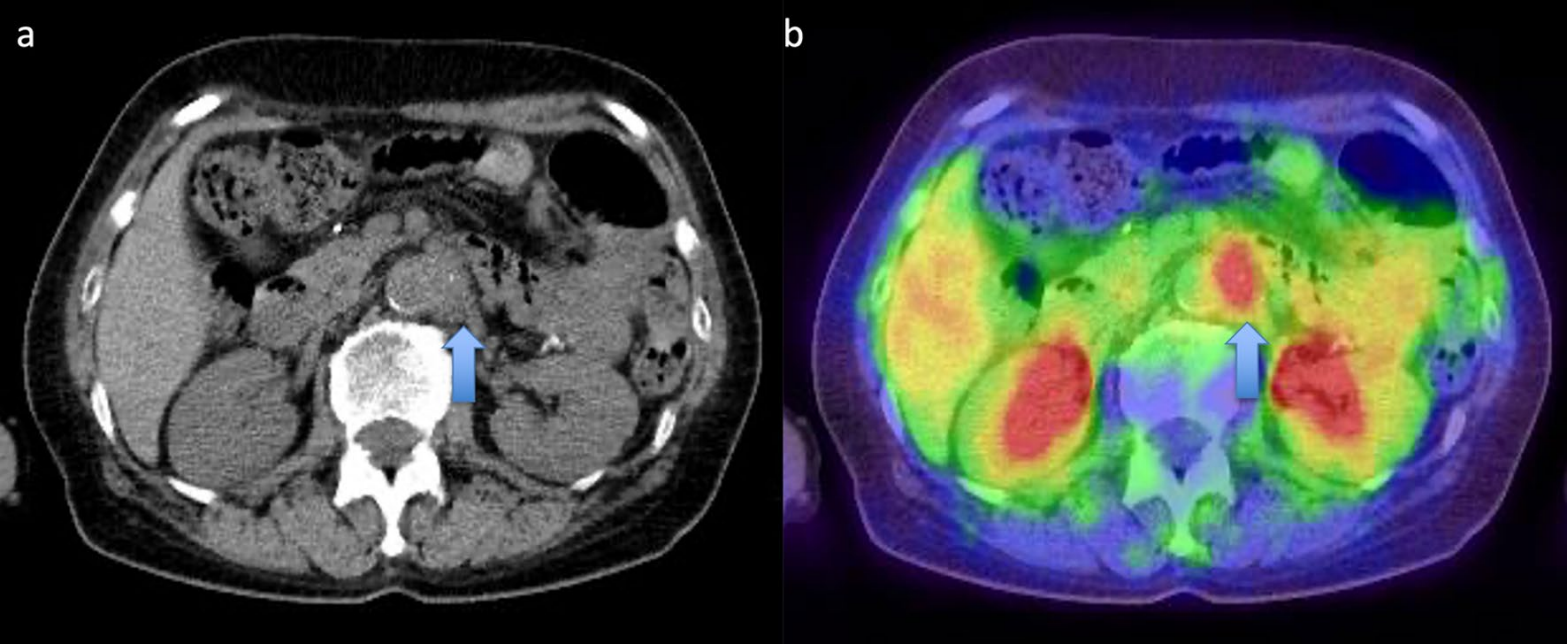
At postoperative year 12, computed tomography (a) / positron emission tomography (b) detected a 30 × 14 mm lymph node with abnormal uptake in the No. 16b1lat lymph node station
